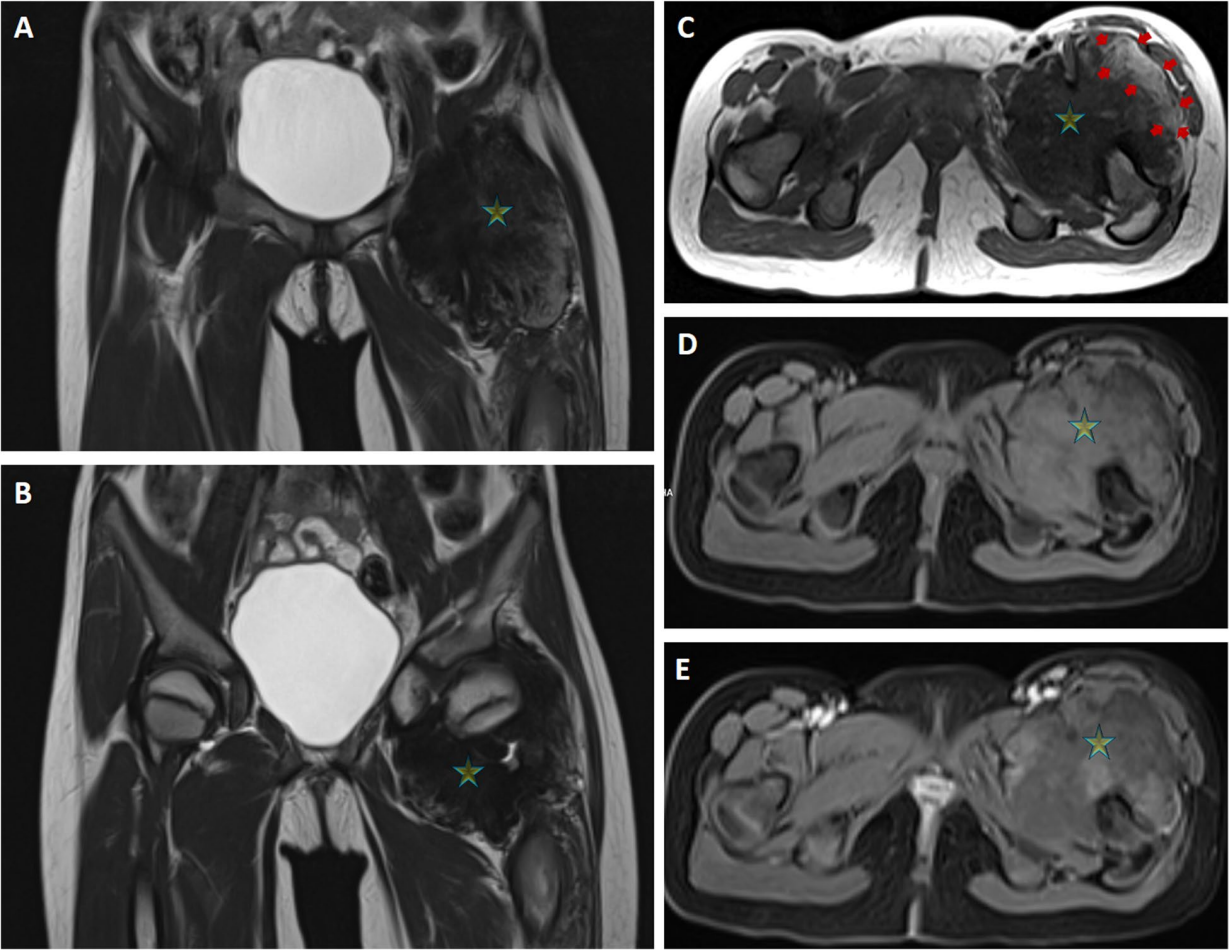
Coronal non-fat-suppressed T2-weighted (A and B) and axial (C) non-fat-suppressed T1-weighted magnetic resonance (MR) images of the left hip show a predominantly hypointense intra-articular mass (star) containing high-signal foci (red arrows) representing a fibrolipomatous component extending into the anterior muscular plane. Pre- and post-contrast fat-suppressed T1-weighted MR images (D and E) show minimal contrast enhancement and reveal signal loss in the fat-containing components of the mass
